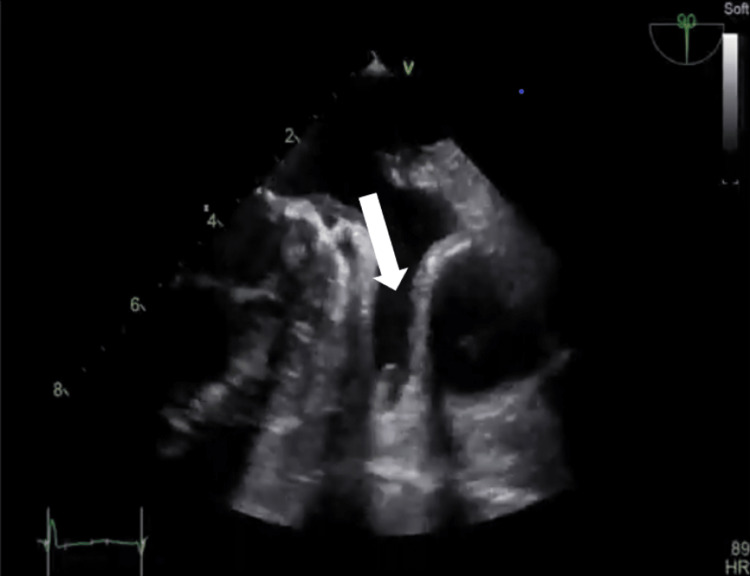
Transesophageal echocardiography focusing on two-dimensional images of the clear left atrial appendage. Note the pectinate muscle that might be mistaken as a thrombus, ruled out using the contrast (arrow).
